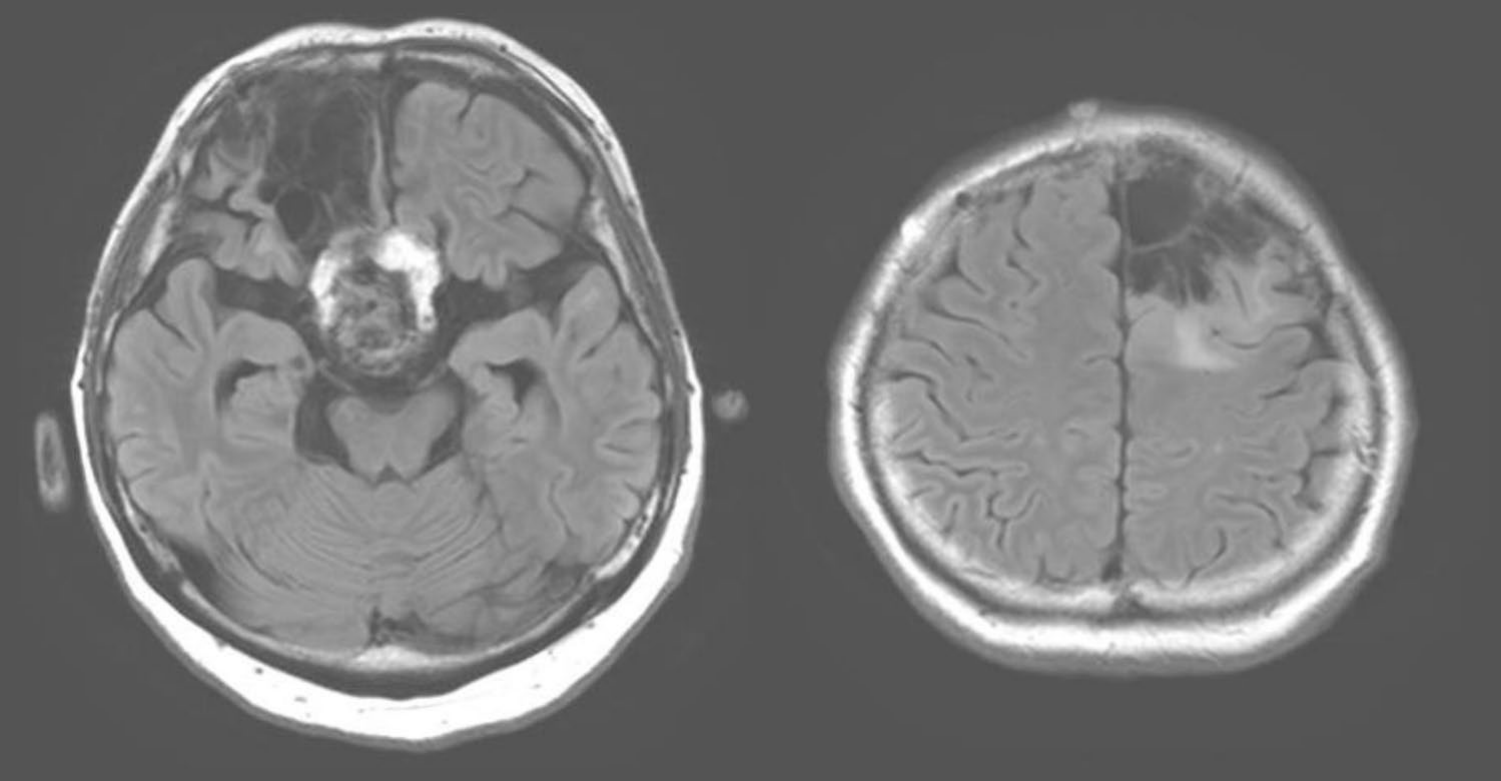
Preoperative FLAIR MRI of the patient. A residual craniopharyngioma lesion and encephalomalacia were observed in the right orbitofrontal region. In the left frontal lobe, postoperative changes and a residual oligodendroglioma lesion were identified along the margin of the resection cavity
